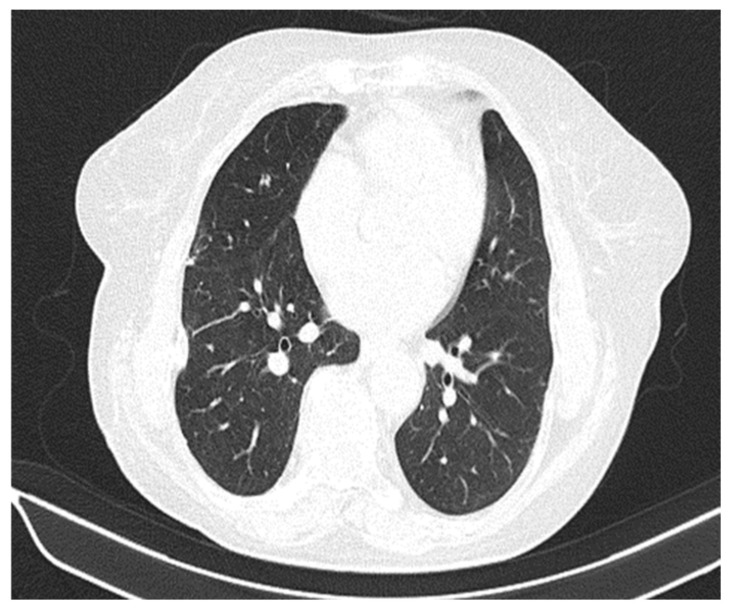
Chest CT performed one year after the first surgical intervention demonstrated a minimal pleura-based area of consolidation. Source: authors’ archive.
